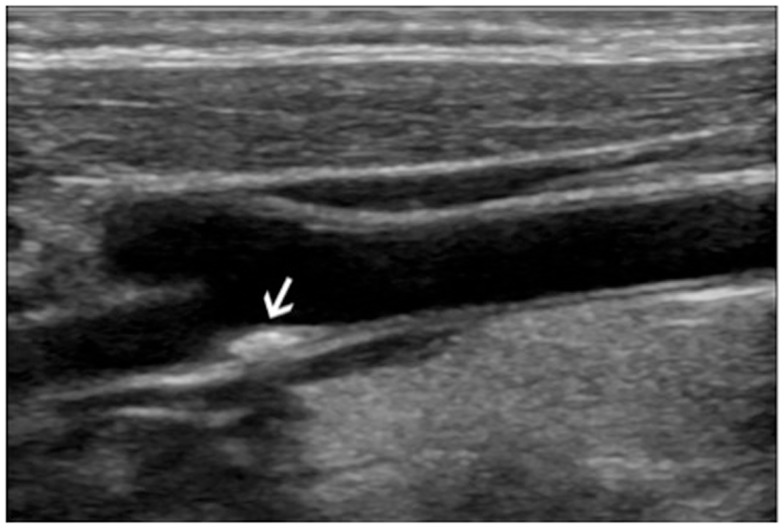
Ultrasound imaging of the right carotid artery bifurcation of a 44-year-old woman with a 10 years history of systemic lupus erythematosus: the arrows show an atherosclerotic plaque extending toward the internal carotid artery.
